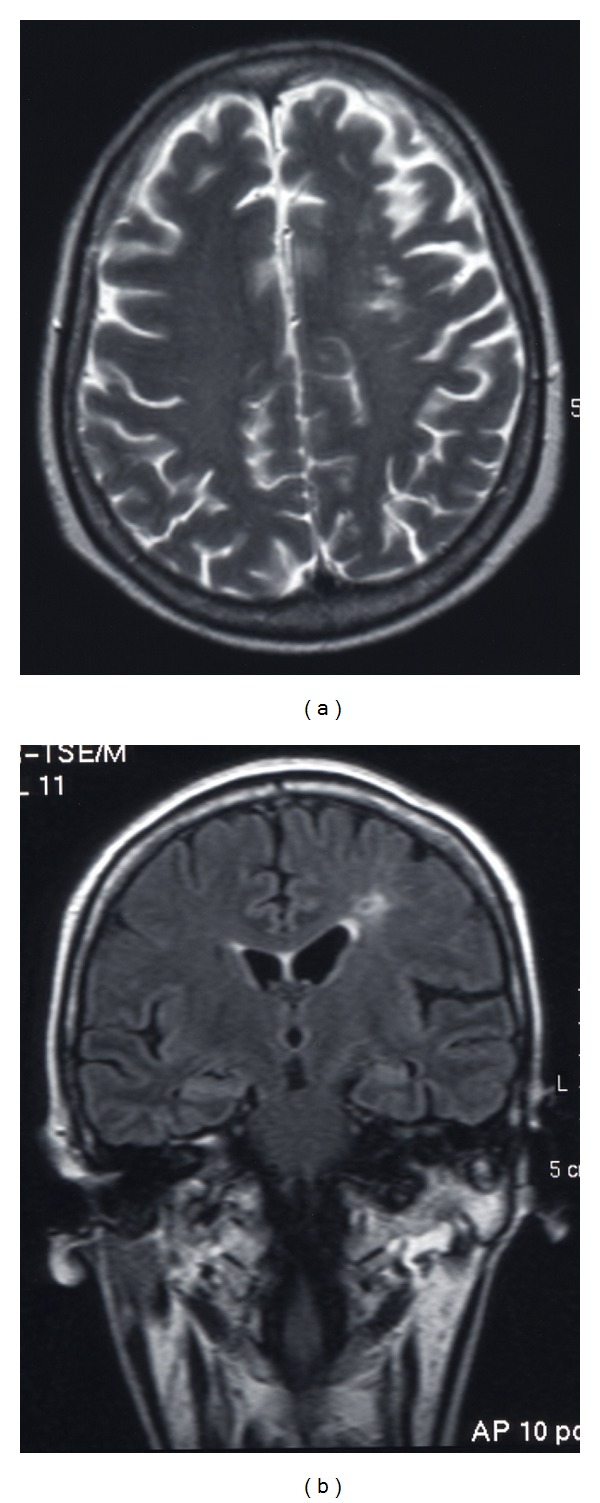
Preoperative magnetic resonance imaging axial T2-weighted image (a) and coronal FLAIR image (b). Cerebral infarction of the left fronto-parietal watershed area was noted.
